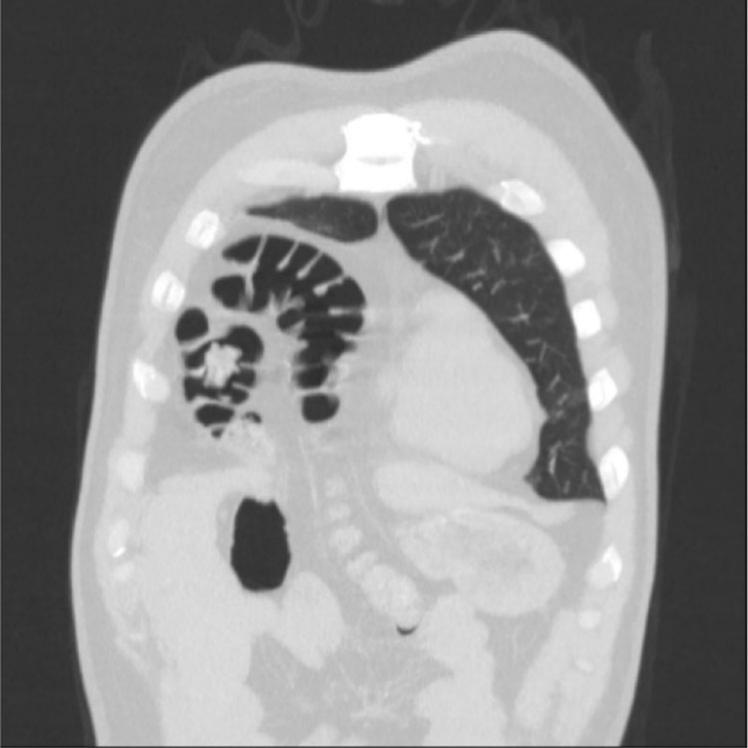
Computed tomography scan of a 41‐year‐old male patient with Morgagni hernia (Coronal cut).
